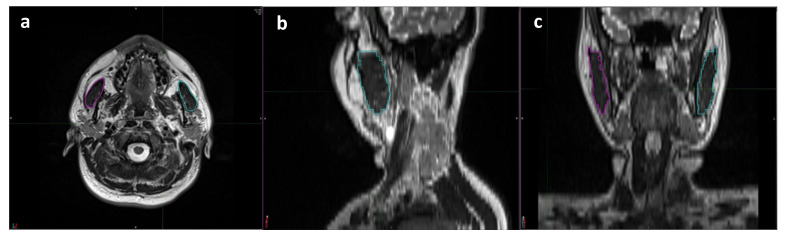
Representative delineation process used for volumetric measurements of each masseter muscle using T2 weighted magnetic resonance imaging scans: (a) axial, (b) sagittal, and (c) coronal planes (magenta: right masseter muscle; cyan: left masseter muscle).
